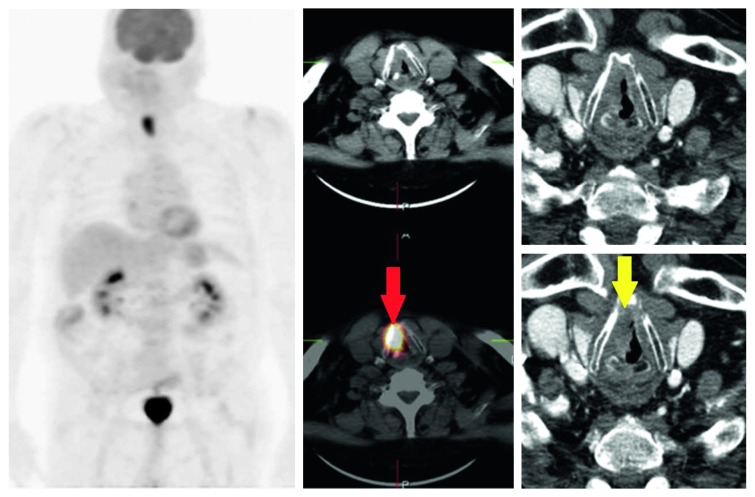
Pt T4a (cartilage infiltration): PET/CT positive (TP, red arrow) vs. CECT negative (FN, yellow arrow). SUVmax, 19.52; MTV, 11.15 cm^3^.
